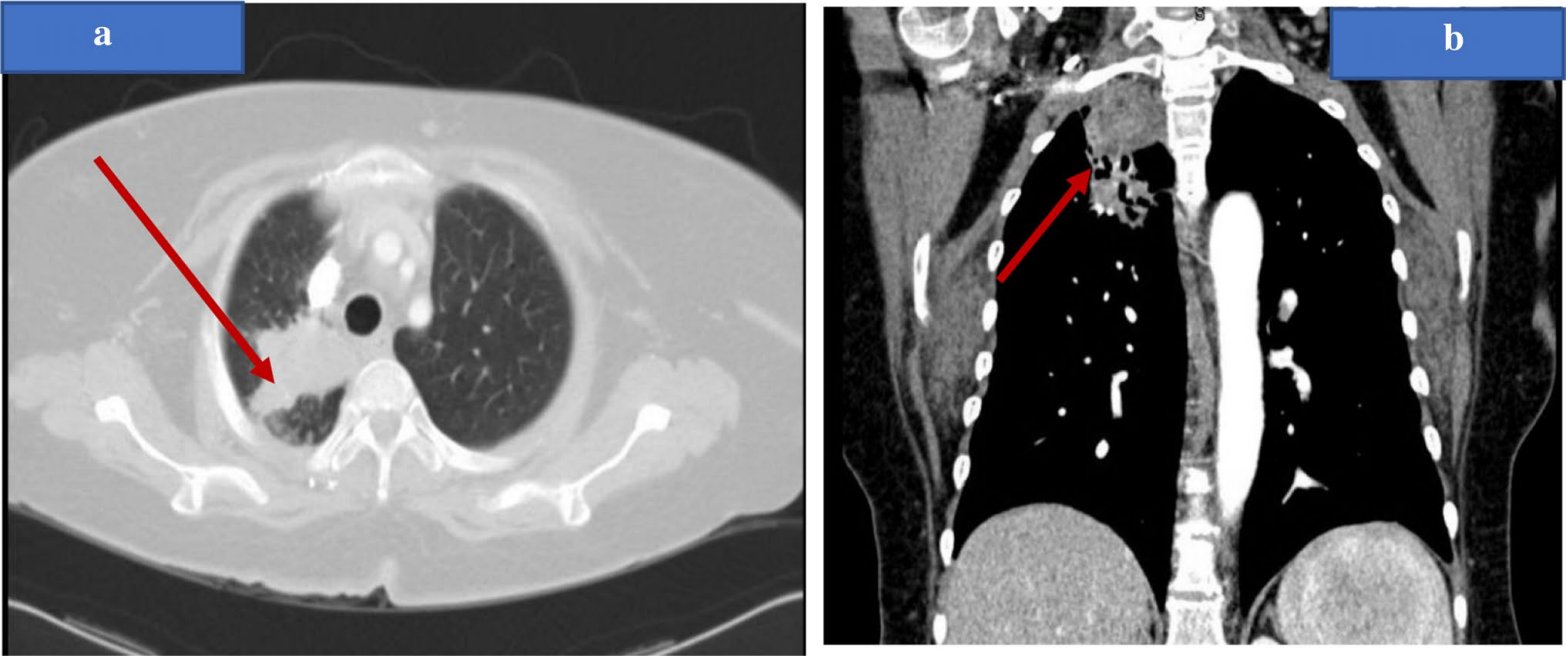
a, b Chest CT showing a well-defined right apical mass lesion with pleural invasion and possible mediastinal extension, suggestive of Pancoast tumor (red arrow)
